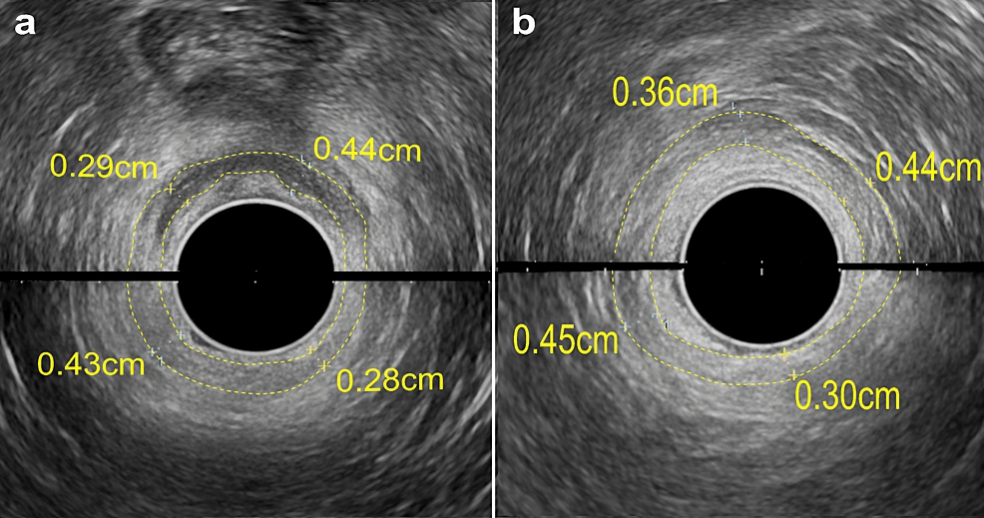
Anal ultrasound images Using a 180-degree probe, each image showed the anterior (top) and posterior (bottom) aspects. Image a shows pre-treatment (T0), and image b shows post-treatment (T18). The yellow dotted lines indicate the internal anal sphincter as detected by ultrasound. Measurements of sphincter thickness at four equidistant points, using the perineum as the 12 o'clock reference and moving clockwise, are provided in centimeters (cm).
